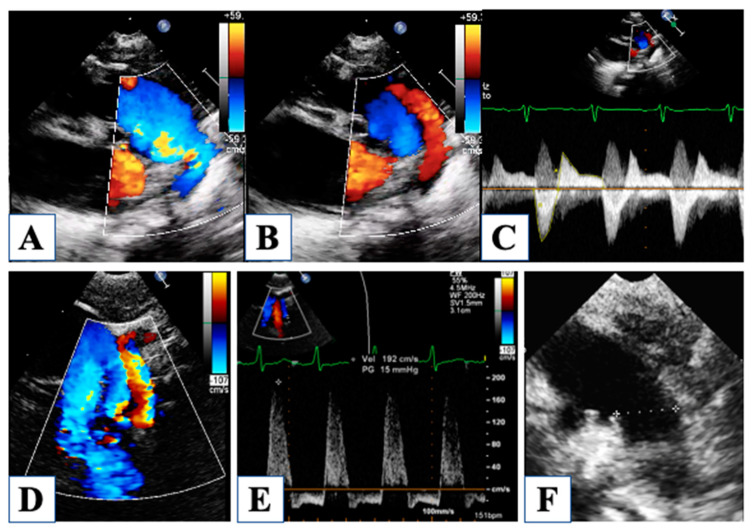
Dilated pulmonary trunk with aortic valve atresia and wide ductus arteriosus providing systemic cardiac output with right-to-left shunt in systole (panel (A)) and left-to-right shunt due to lower pulmonary compared to systemic resistances (panel (B)). Continous-wave Doppler through the ductus allows measurement of the amount of flow in each direction by tracing the Doppler curves (Panel (C)). Panel (D) shows a diminutive takeoff of the aortic arch from the ductus, generating increased velocity of the reversed aortic flow measured by Pulsed-wave Doppler (Panel (E)). Ductus arteriosus can be very large compared to pulmonary arteries, as shown in panel (F).
